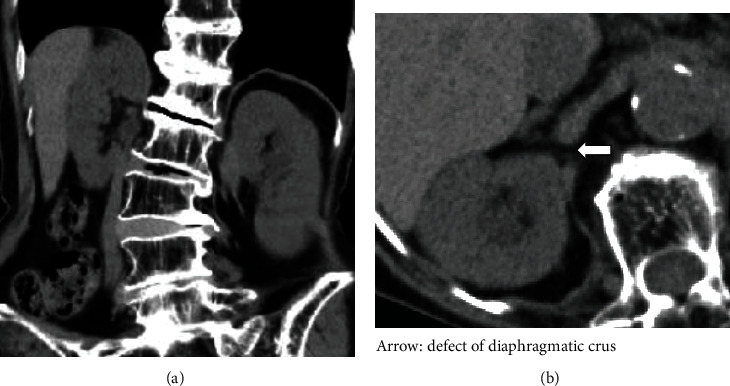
Previous CT of the abdomen revealed the right kidney in a higher position than the left one ((a) coronal) and a defect of the right diaphragmatic crus which eventually became the hernia orifice ((b) axial).
